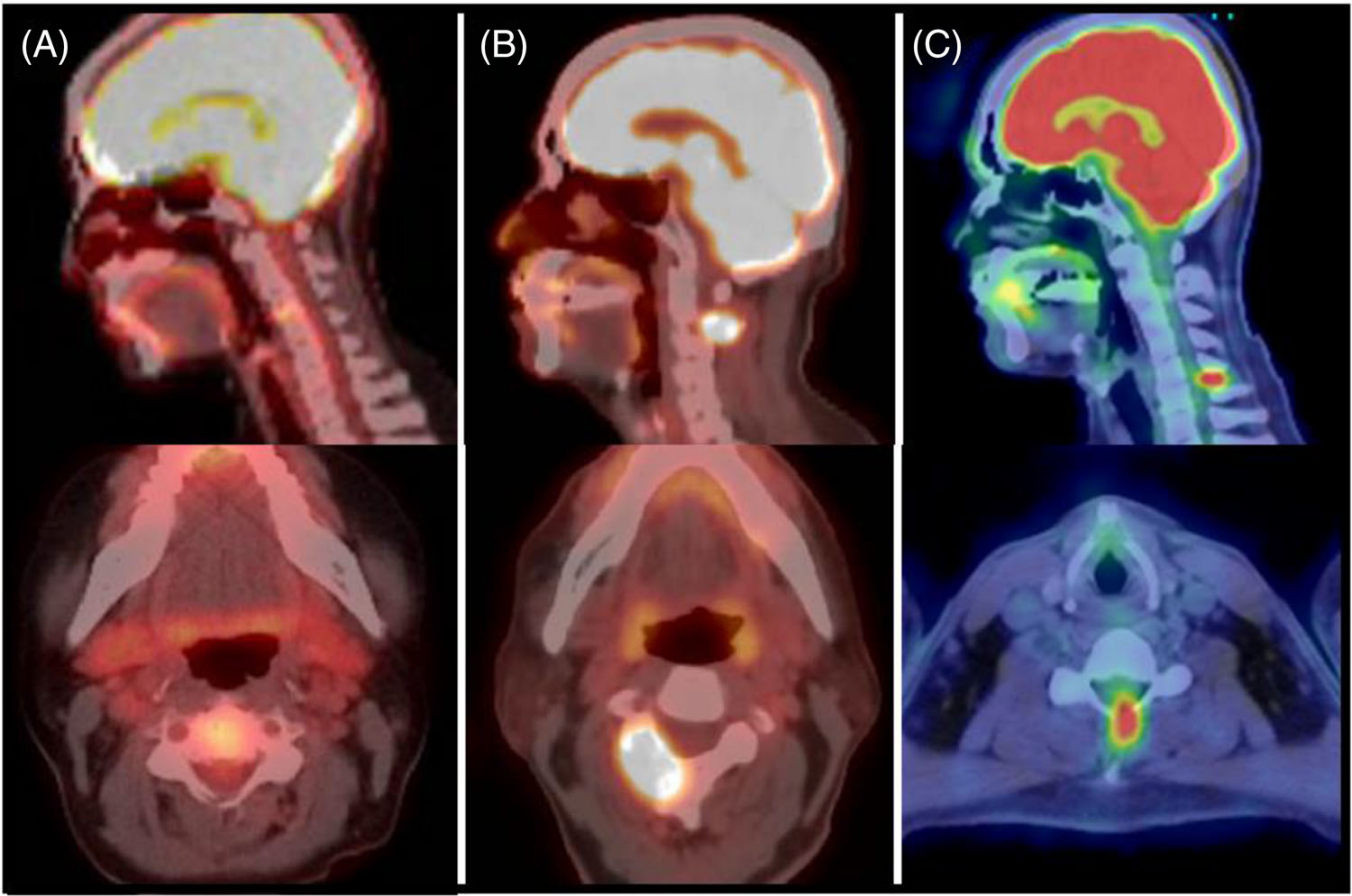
Positron emission tomography/computed tomography (PET/CT) images of three Langerhans cell histiocytosis (LCH) cases with cervical spine involvement
